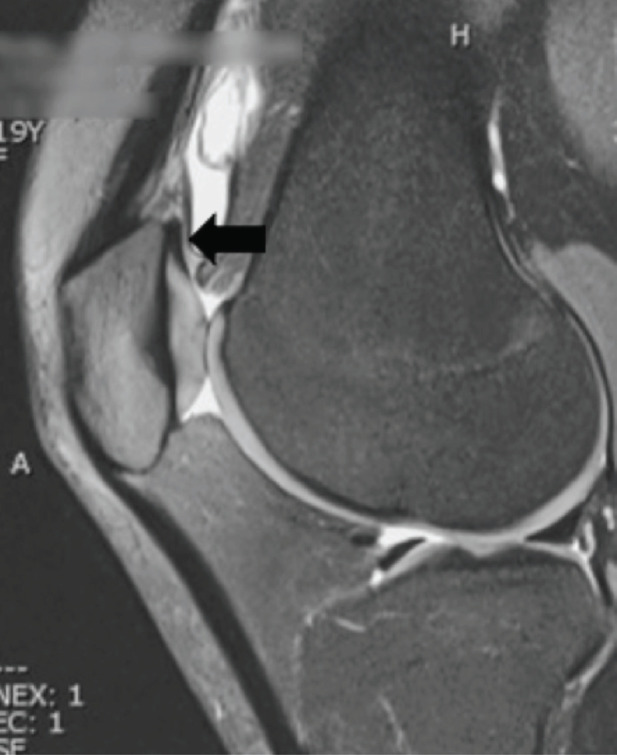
MRI imaging. Complete suprapatellar plica (arrow) preventing communication between the sub quadricipital recess and the rest of the joint space.
